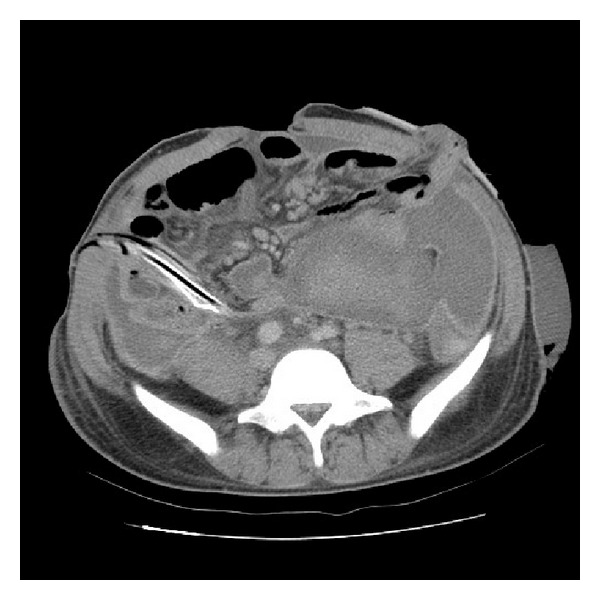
Due to persistent fluid discharge postoperatively, further abdominal CT revealed fluid collection within the abdominal cavity. A percutaneous drainage was inserted and massive pus-like fluid was drained.
